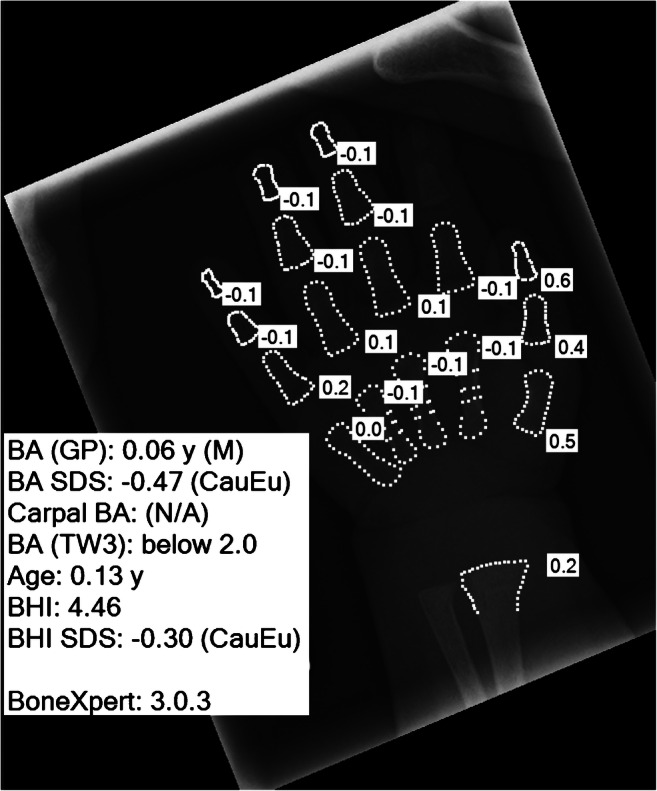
Posteroanterior left hand and wrist radiograph in a 3-week-old boy, following interpretation of bone age by BoneXpert. The figures in the small white boxes represent the Greulich and Pyle bone ages of the individual bones. *BA (GP)* Greulich and Pyle bone age (gender), *BA SDS* Bone age standard deviation score (ethnicity), *BA (TW3)* Tanner and Whitehouse three-bone age, *BHI* bone health index (digital X-ray radiogram), *BHI SDS* bone health index standard deviation score (ethnicity), *CauEu* Caucasian European, *M* male, *N/A* not available (no ossified carpal bones in this child), *y* years. See also Table 2
